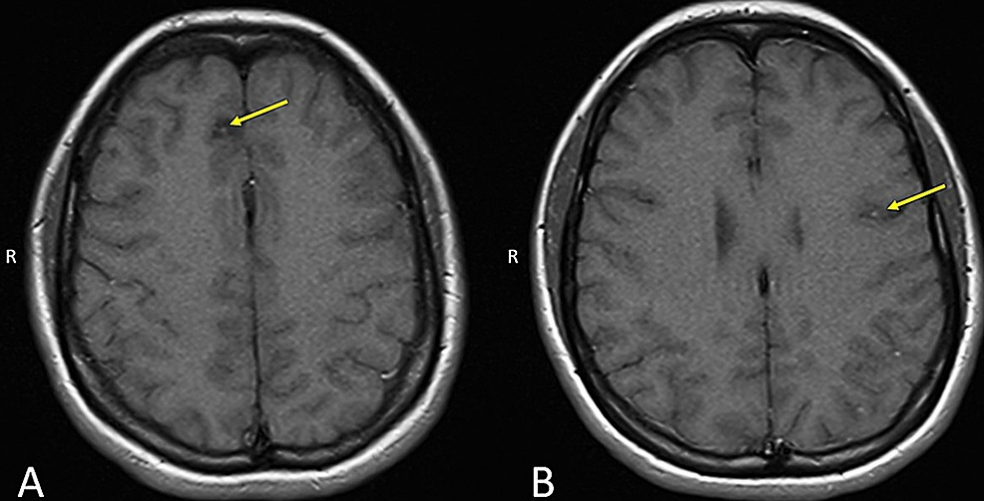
Compared to initial MRI findings (Figure 2), all six cysticerci on repeat MRI displayed a decrease in overall size and contrast enhancement after initiating antiparasitic treatment. This can be seen here in the right frontal (A) and left parietal lobes (B) cysticerci (yellow arrows).
